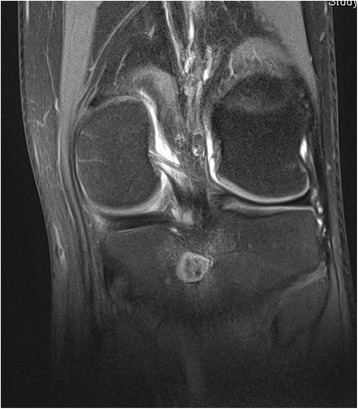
Magnetic resonance imaging scan of the left knee (coronal view), follow-up examination (3 months postoperative)
